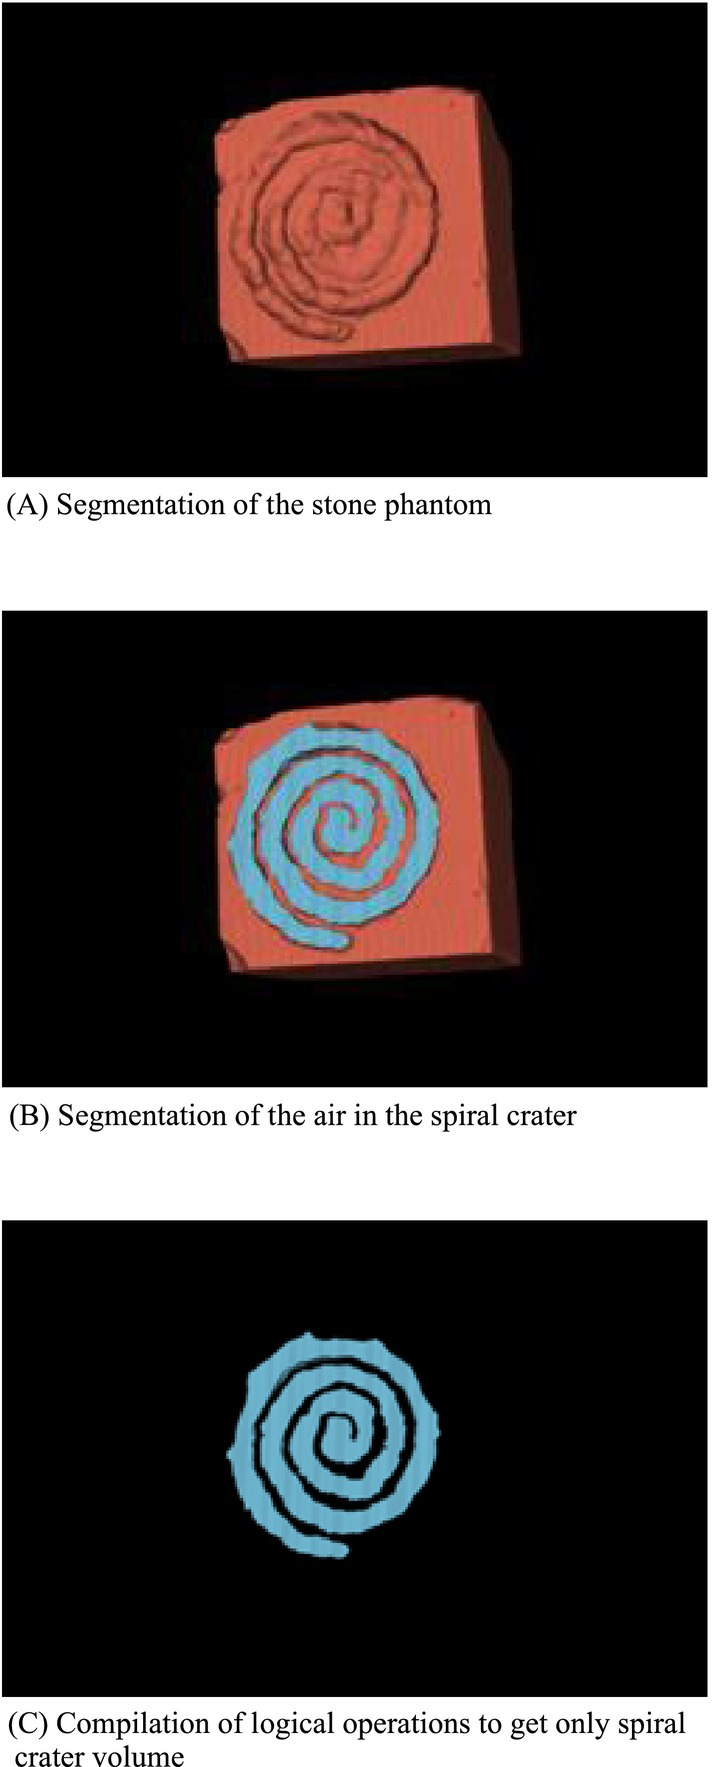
Segmentation process using 3DSlicer to assess ablation volumes based on DICOMs' non‐enhanced computed tomography. First, segmentation of the stone (a), then segmentation of the air of the spiral crater (b) and compilation of both to get the ablation volume (c).
